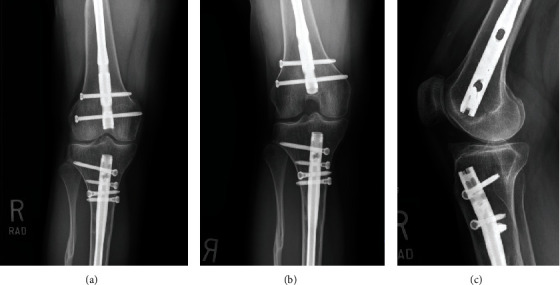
Preoperative right knee radiographs demonstrate retrograde femoral rod fixation and antegrade tibial rod fixation: (a) anterior-posterior view; (b) posterior-anterior flexion view; (c) lateral view, which best demonstrates the anterior intra-articular heterotopic.
